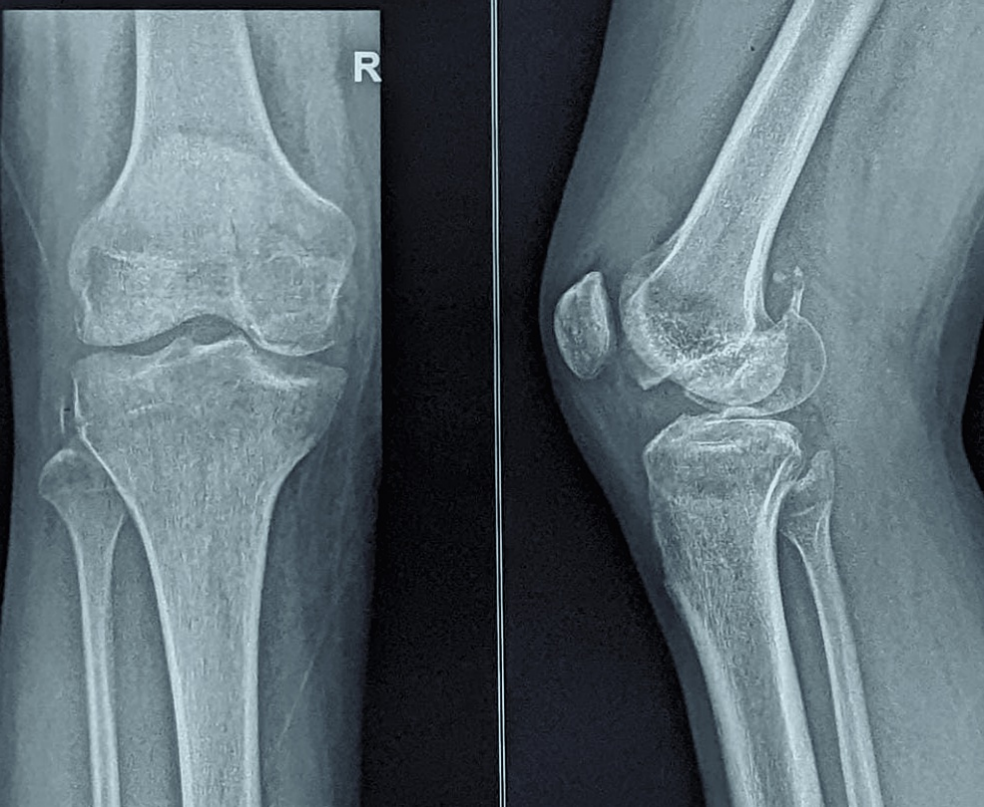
Plain radiograph of the right knee in anteroposterior and lateral view showing the Hoffa fracture of the medial condyle distal femur
